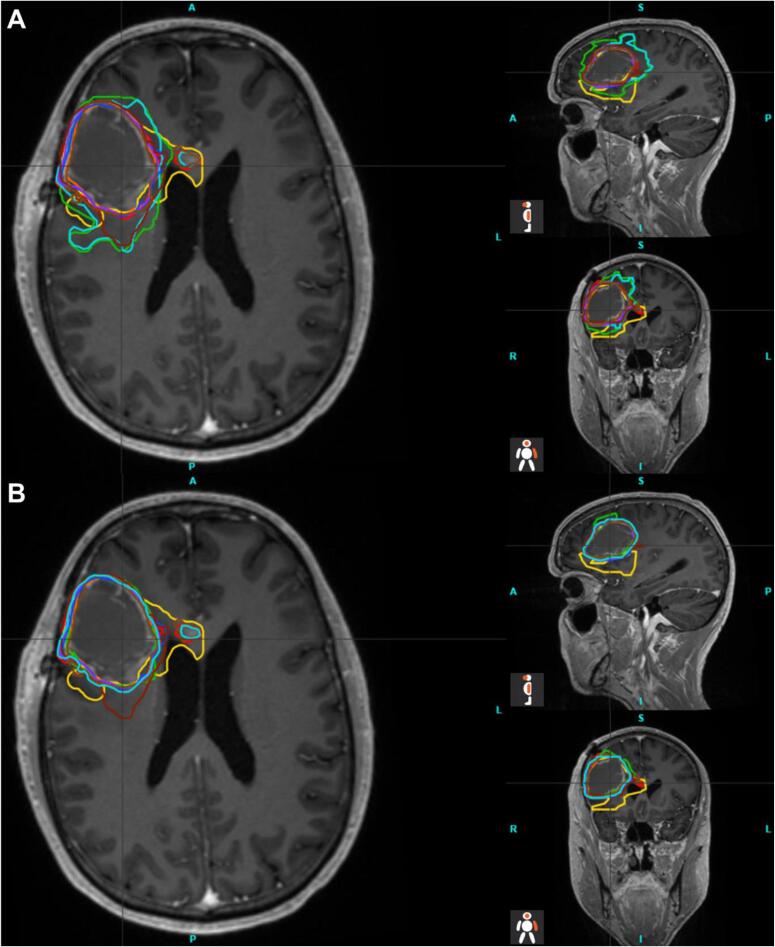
MRI images including the structure sets from all study sites for the GTVu, first round (A) and after correction(B). (*A = anterior; P = posterior; S = superior; I = inferior; L = left; R = right).*
